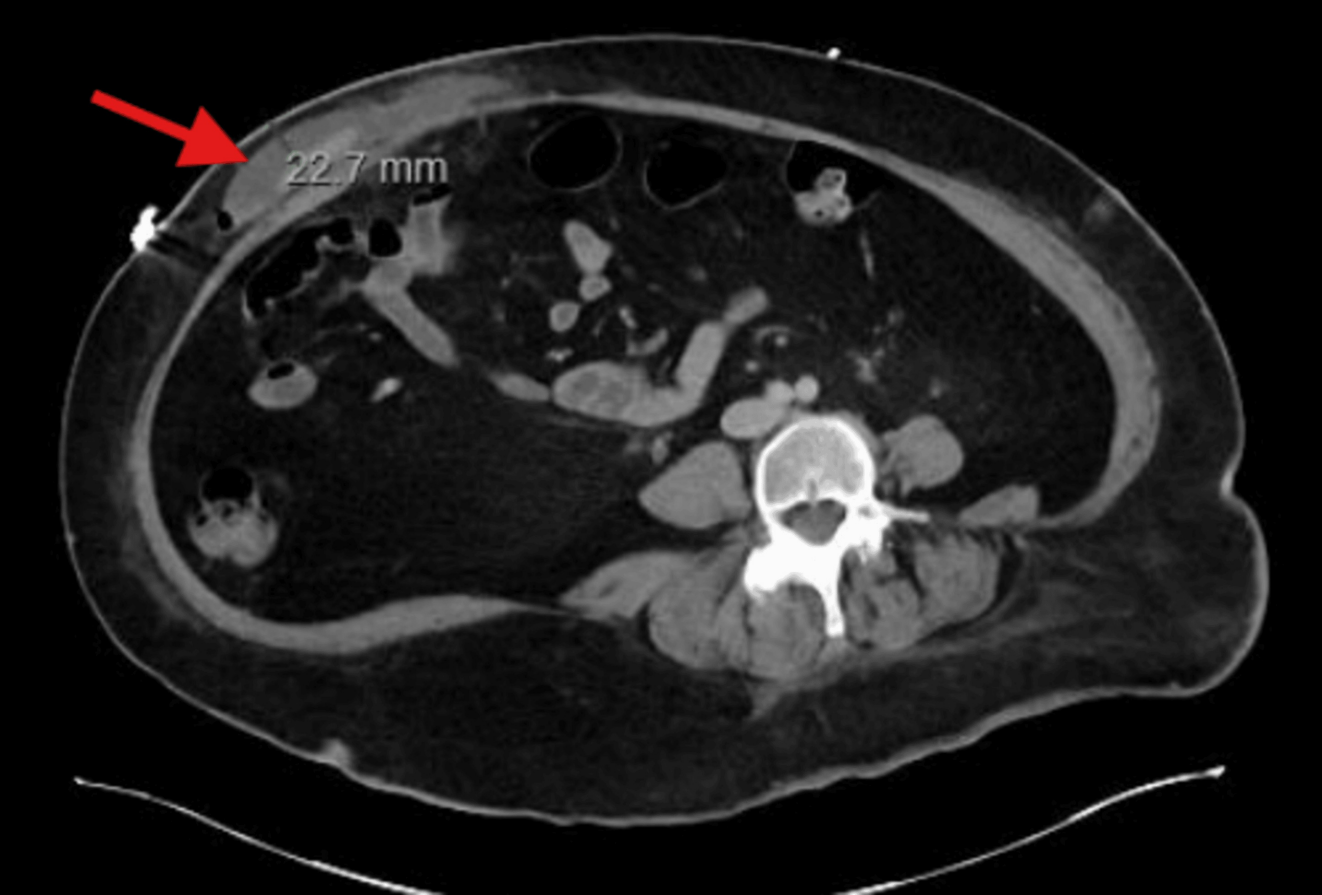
CT of abdomen and pelvis with contrast showing an approximately 2 cm mixed density fluid collection with small internal foci of air involving the right anterior lateral abdominal wall that is marked with a red arrow
